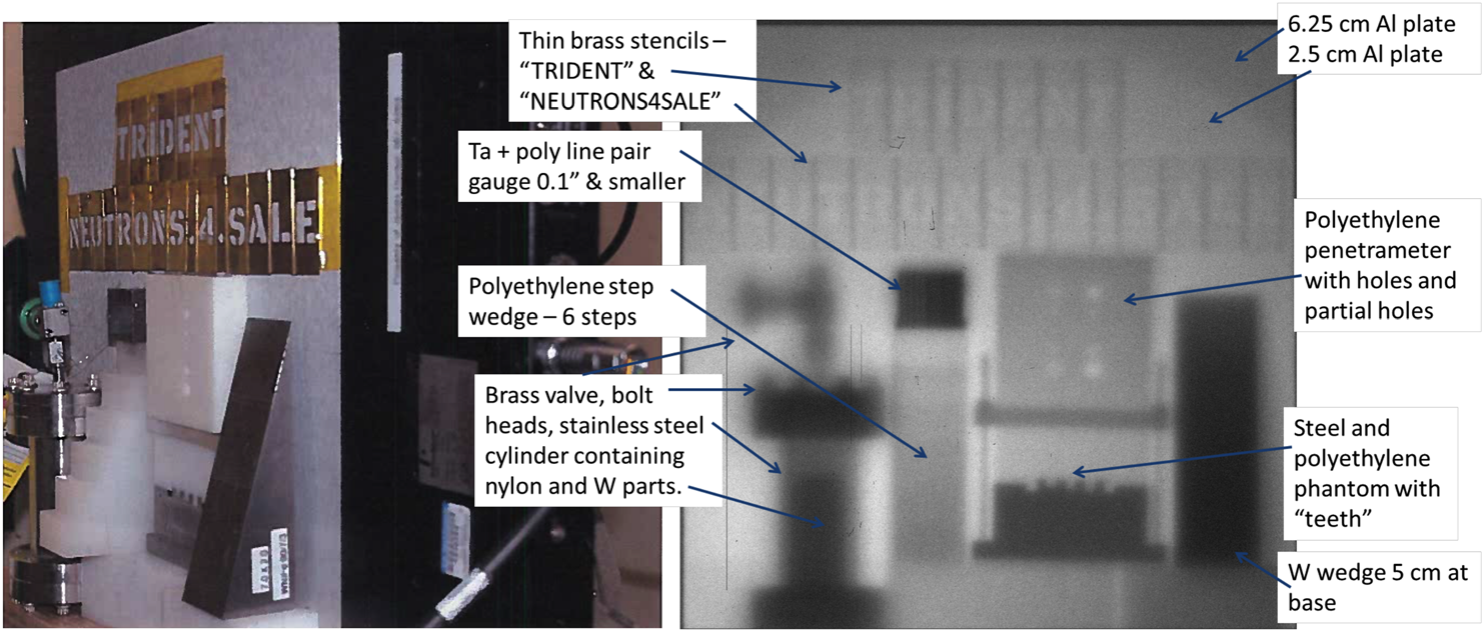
Radiography of thick objects with the laser-driven neutron-source at Trident.
